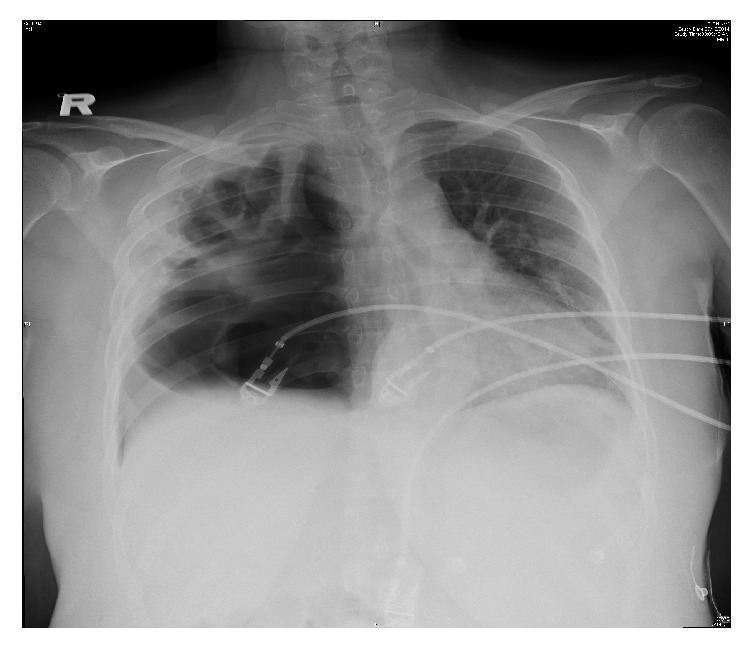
Chest X-ray showing right upper lobe cavitary lesion with a large pneumothorax and pleural effusion.
